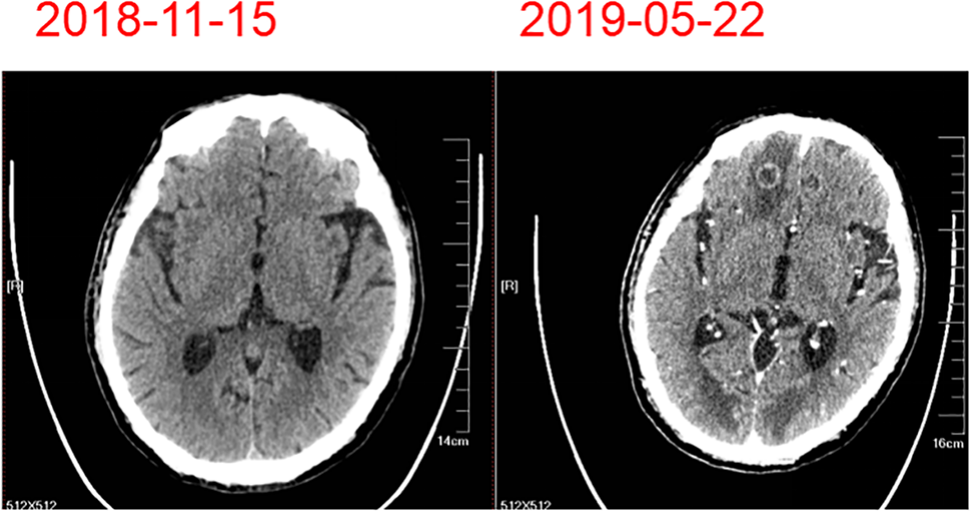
Brain masses observed by brain computed tomography before and after treatment.
